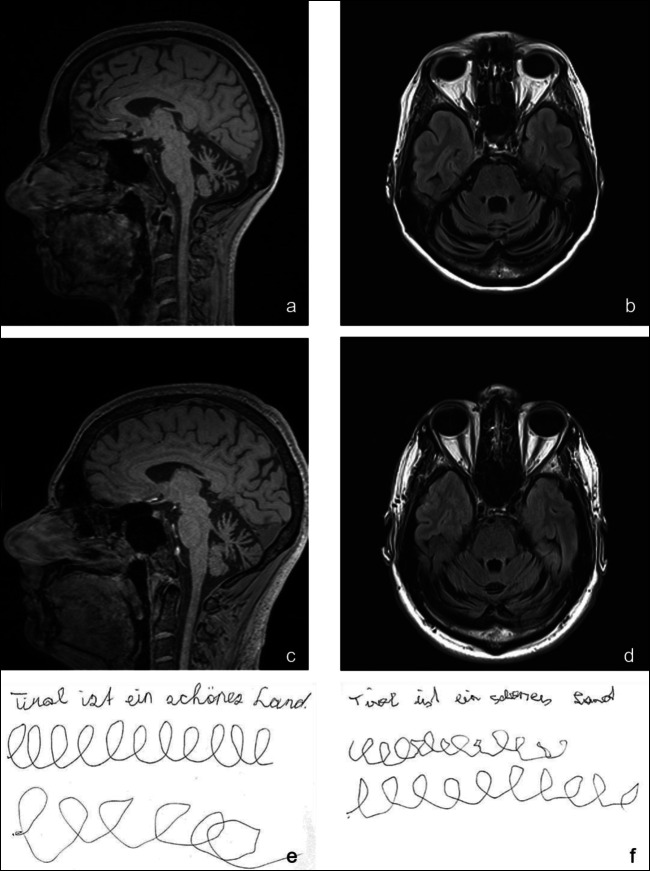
Sagittal and axial MRI imaging demonstrating cerebellar atrophy in the index patient (a and b) and patient III-3 (c and d). Writing samples depicting writer’s cramp associated dysgraphia (full sentence and first L-letter series written with dominant hand, second L-letter series drawn with non-dominant hand) in the index patient (e) and patient III-3 (f)
